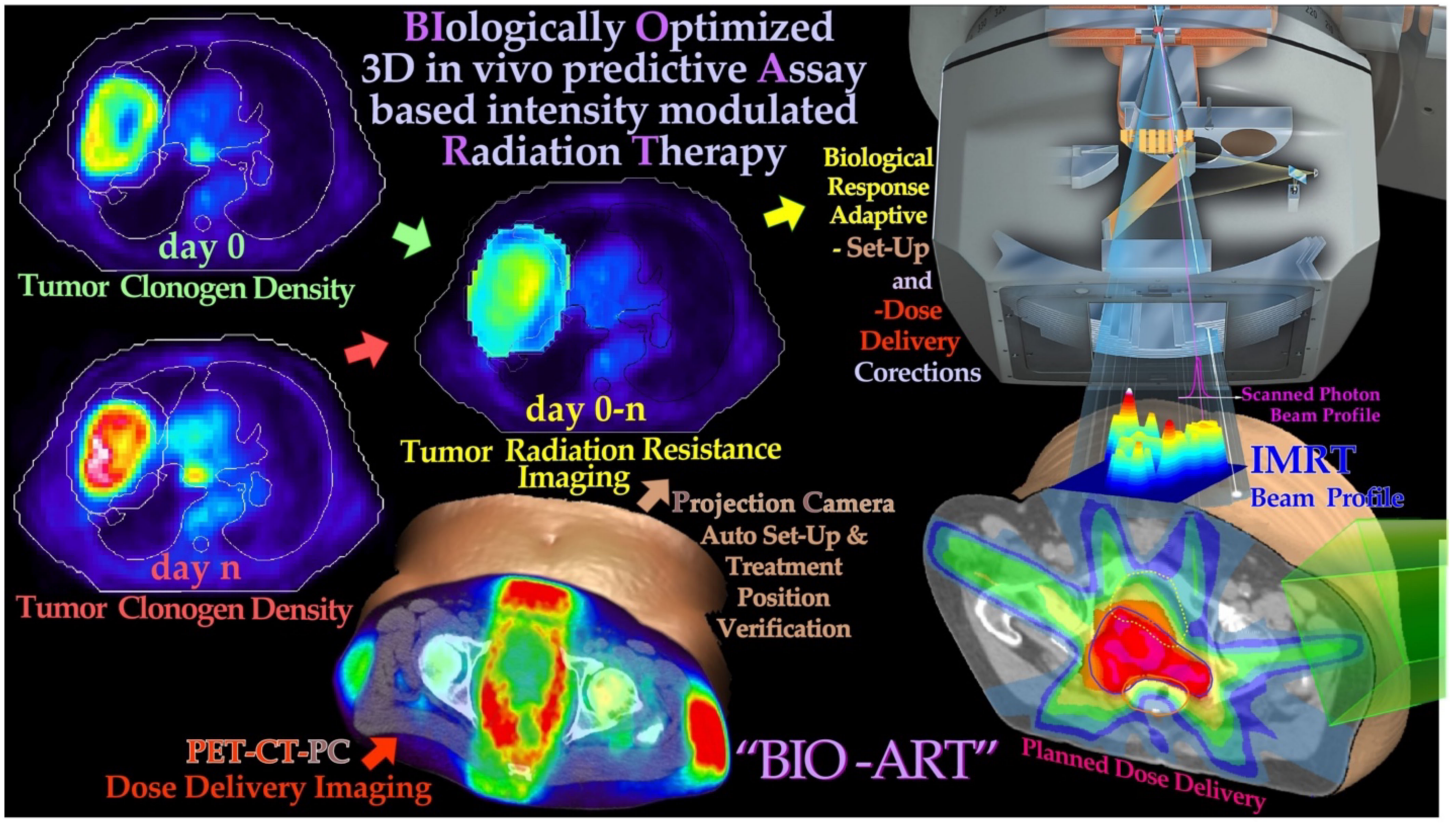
PET-CT-PC can be used to derive tumor responsiveness information that can be used to plan, verify, and biologically optimize using the *in vivo* predictive assay-based radiation therapy method [BIOART (20, 27, 70)]. By measuring the tumor cell kill early in the treatment, 3D *in vivo* predictive assay information about radiation responsiveness is obtained for accurate prediction of the optimal dose delivery based on measured hypoxic tumor cell response status and adaptive control (4, 11, 27). Different imaging methods from various body regions exemplify aspects of the adaptive feedback procedure (4). The normal tissue responses are approximately known from historical data (20, 27). Both with light ions and scanned high-energy photon beams, 3D *in vivo* PET-CT dose delivery monitoring is possible to further optimize the treatment based on observed mean dose delivery, which may differ from the planned dose delivery without considering organ and patient motion. Both these data sets when used together will allow a high degree of therapy optimization where practically all major sources of treatment error can be picked up as long as they influence tumor cell survival and can thus be corrected for using biologically optimized adaptive treatment techniques [cf [2].: Figure 126, right panel, where the lower part of the tumor was under-dosed by organ motion in and out of the beam with increased cell survival in the lower part of the tumor (11, 23, 27)]. Modified from [7: Figure 125].
